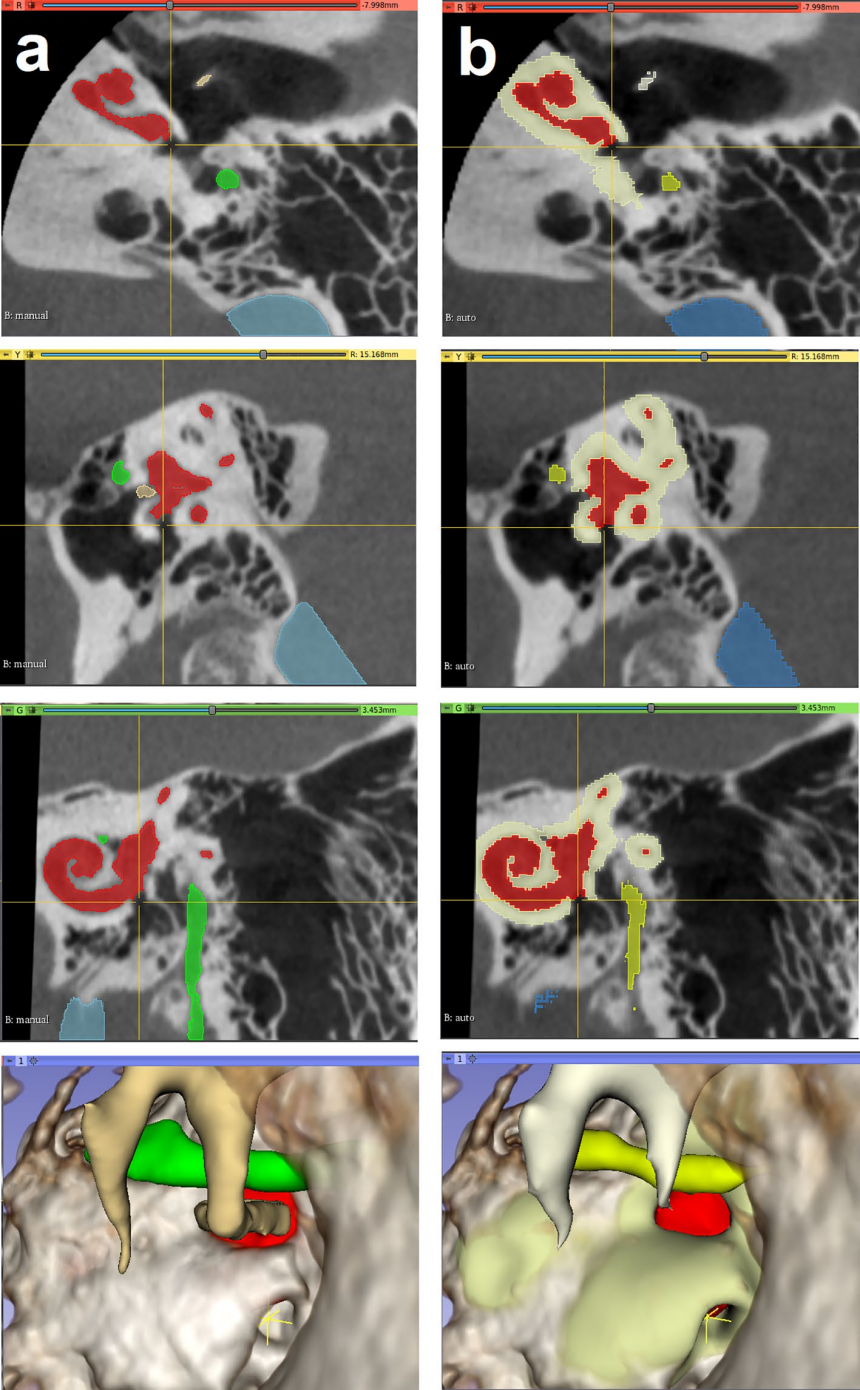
Round window niche (Crosshair) from a manual labeled scan (left—a) side by side with the auto-segmented CT dataset (right—b). From top to bottom, windows from 3D Slicer showing the axial, sagittal, coronal and 3D rendered view of the middle ear. Inner ear (red), ossicles (ivory); facial nerve (yellow), sigmoid sinus (blue) and otic capsule (green).
